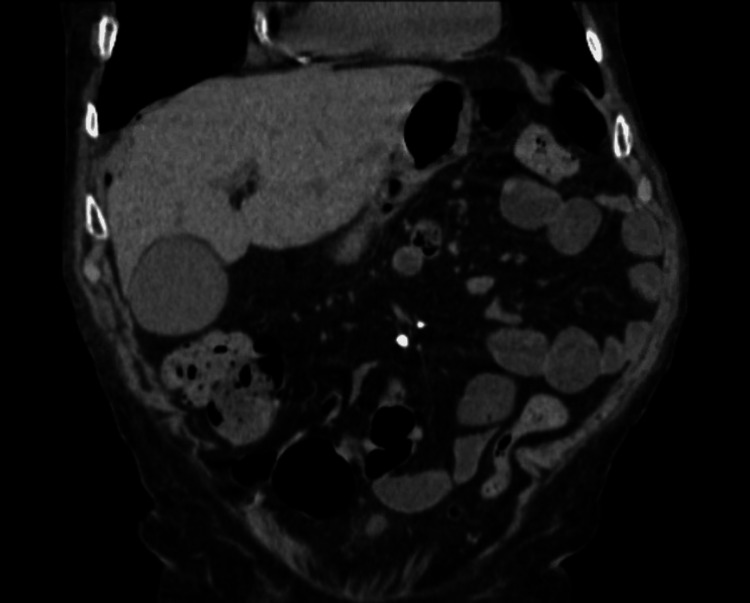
CT abdomen/pelvis showing resolving fluid collection in the stomach and decreased pneumoperitoneum
